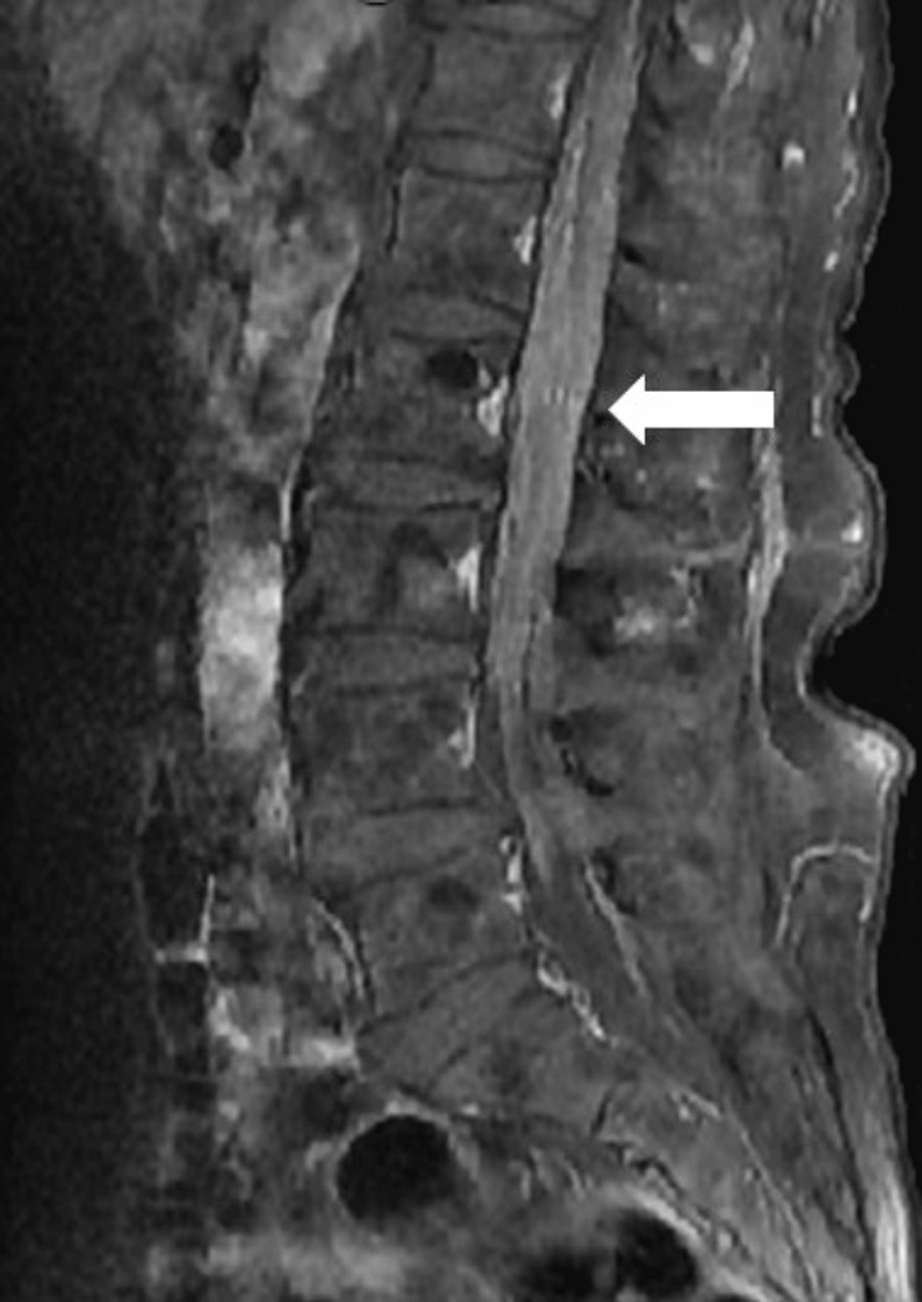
Lumbar MRI with contrast showing leptomeningeal enhancement of the cauda equina. MRI: magnetic resonance imaging.
